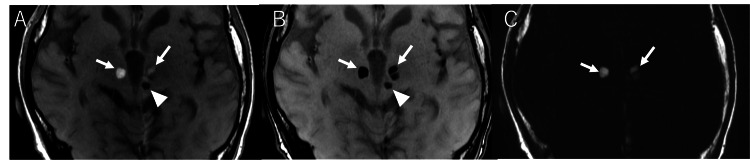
Chemical shift imaging on MRI. In chemical shift imaging using the Dixon method, the bilateral thalamic lesions show signal reduction on out-of-phase images (A: arrows) compared to in-phase images (B: arrows), whereas the left cerebral peduncle lesion does not show signal reduction (A and B: arrowheads). Fat images demonstrate high signal intensity in the bilateral thalamic lesions (C: arrows).
